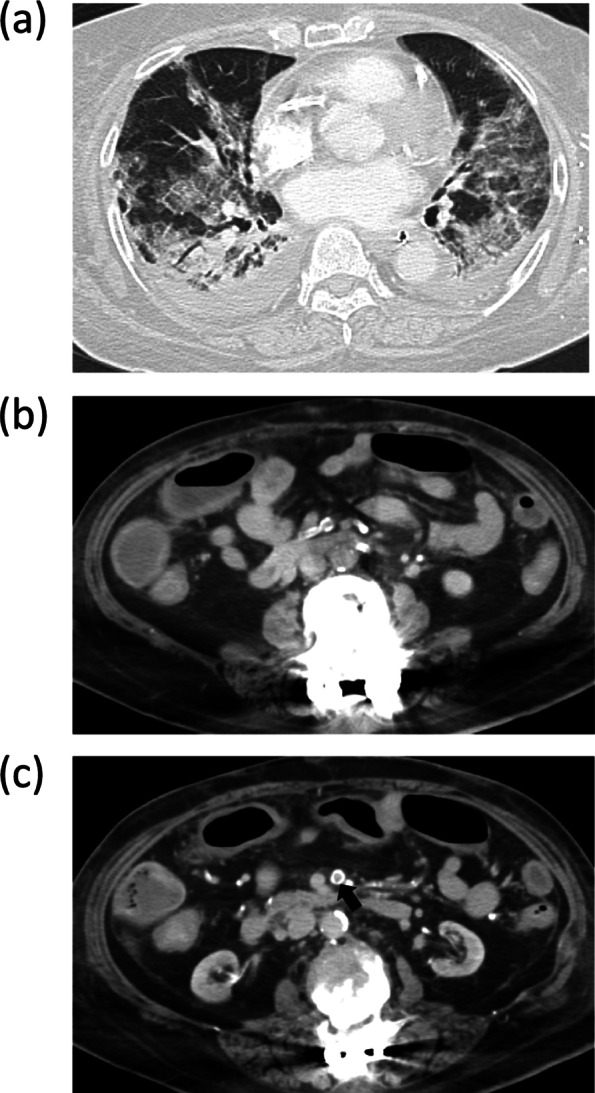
Computed tomography on admission showed bilateral ground-glass opacity with lower-lung predominance (a). There were no obvious abnormal findings in the abdominal region or thromboembolism. The intestine, including the transverse colon was edematous (b). The superior mesenteric artery showed strong sclerotic changes (c, arrow)
